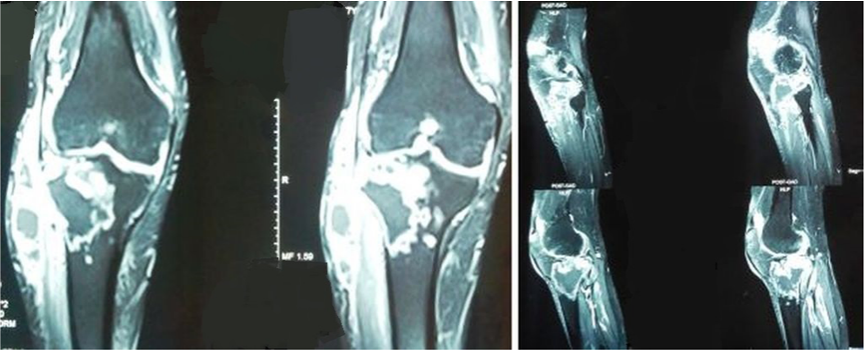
Magnetic resonance imaging of right knee. Magnetic Resonance Imaging of the right knee joint showing intra-osseous and extra-osseous abscesses with synovial inflammation involving knee joint and supra-patellar bursa.
